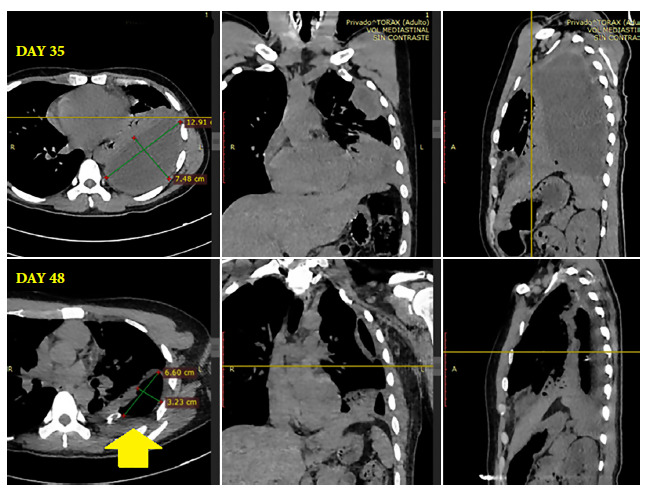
Computed tomography images. Axial view with sagittal and coronal reconstruction, on day 35 of illness shows a liquid collection of homogeneous content, with axial diameters of 12.9 x 7.5 cm and surrounded by a thick wall located, for the most part, in the posterior region. The image on day 48 of hospitalization shows an asymmetric, thick-walled cavity, with axial diameters of 6.6 cm x 3.2 cm, with a hydro-aerial level (yellow arrow), with decreased empyema volume, and adjacent pleural thickening.
